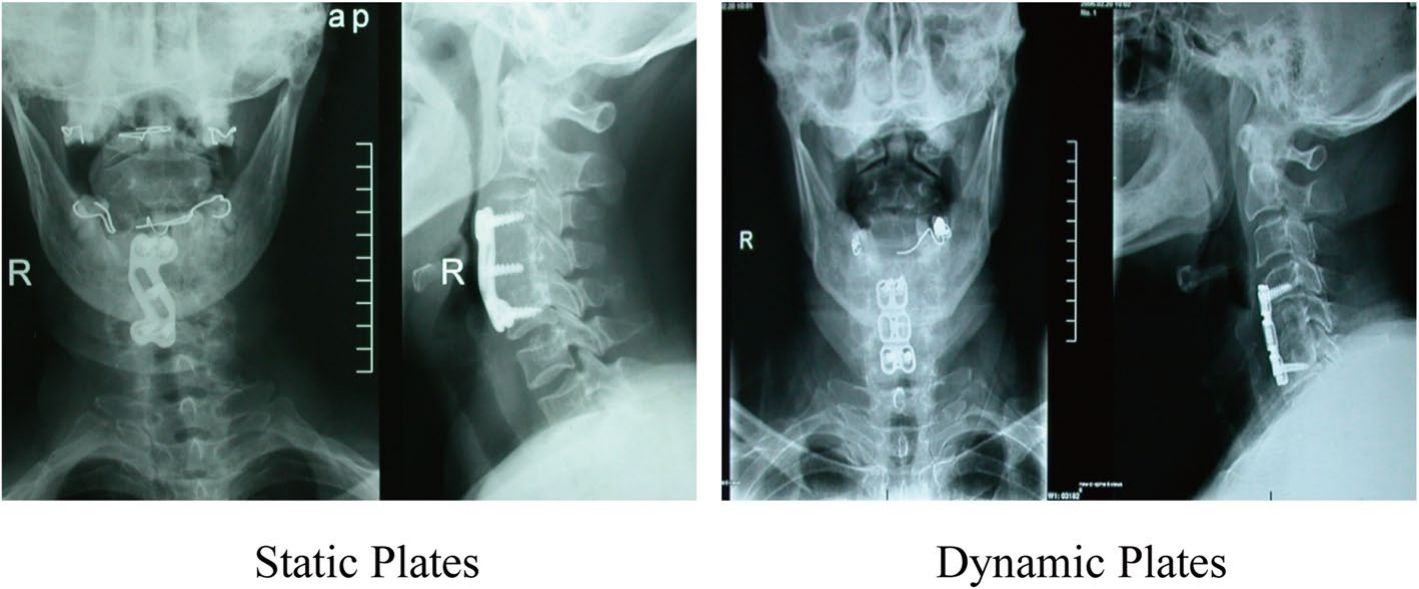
X-ray view of anterior cervical corpectomy and fusion post operation 2 years
